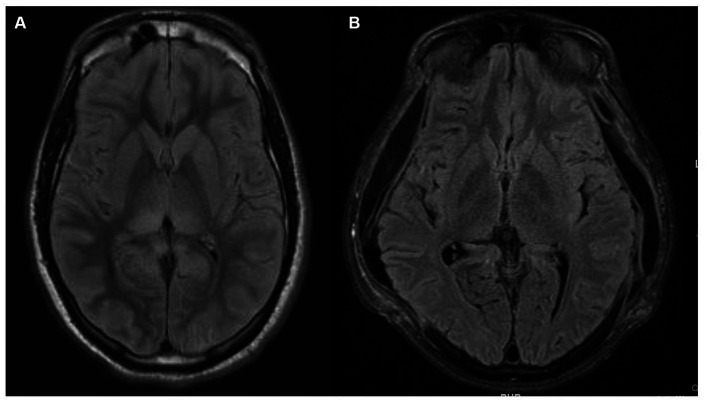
Magnetic resonance imaging (MRI) brain fluid-attenuated inversion recovery (FLAIR) sequences. (A) MRI brain FLAIR sequence that demonstrates bright T2/FLAIR signal, suggesting diffuse grey matter edema which could indicate hypoxic-ischemic brain injury. (B) Repeat MRI brain FLAIR sequence a month later, showing near complete resolution of prior abnormalities and expected extra-cranial herniation of brain through the craniectomy sites.
